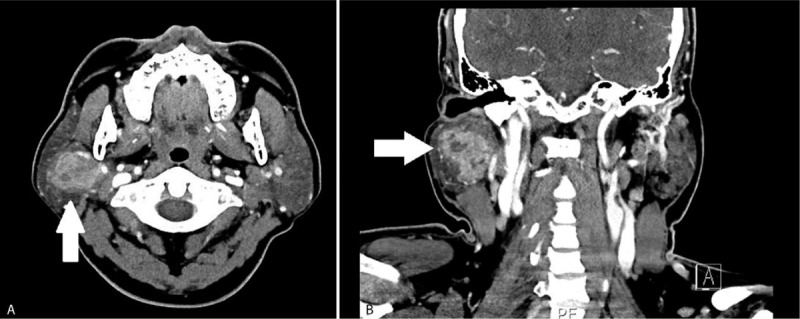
A and 2B. Neck CT revealed about 4.0 cm sized irregular marginated heterogenous enhancing mass in right parotid gland (the white arrow).
